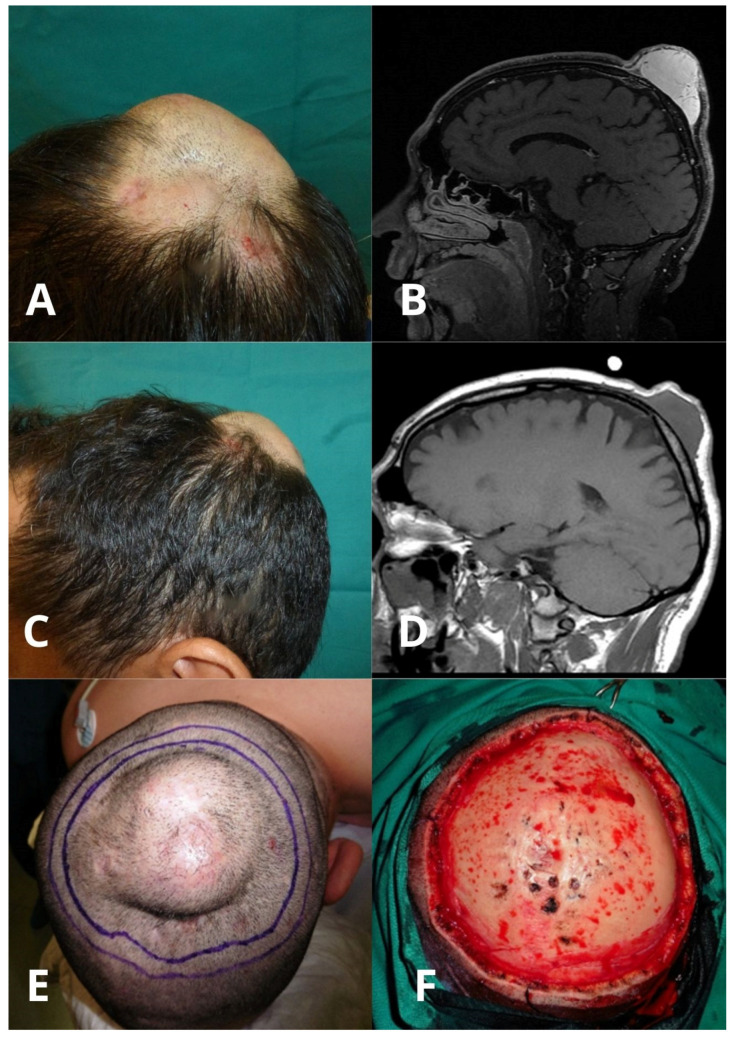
Extensive DFSP on the scalp. Clinical (A) and MRI (B) before imatinib, and clinical (C) and MRI (D) imaging after 3 months of imatinib therapy, with visible tumor volume reduction. (E) Surgical planning for the first MMS stage. (F) Final surgical defect with signs of tumor compression of the calvaria.
